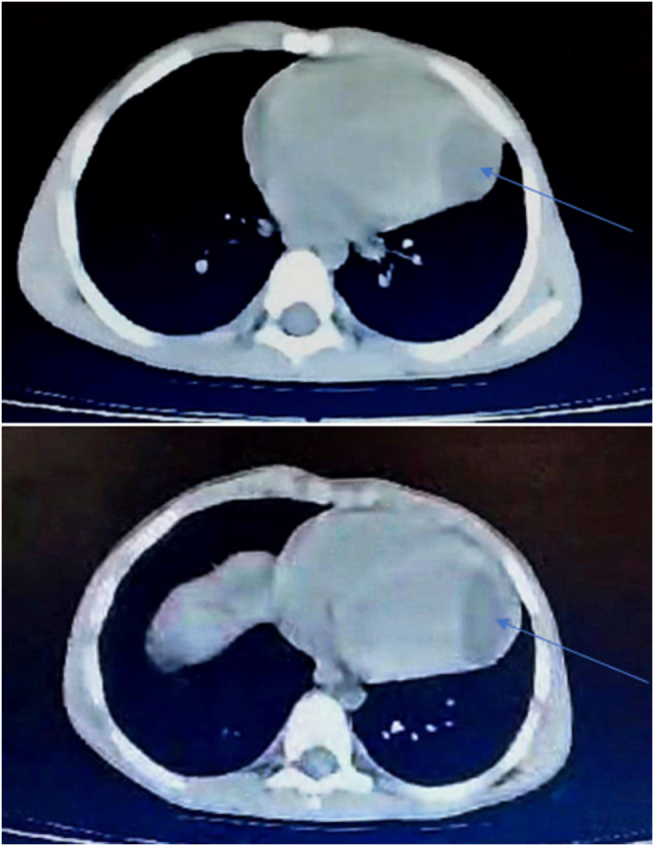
CT image showing the cardiac hydatid cyst and hepatic hydatid cyst (arrow).
